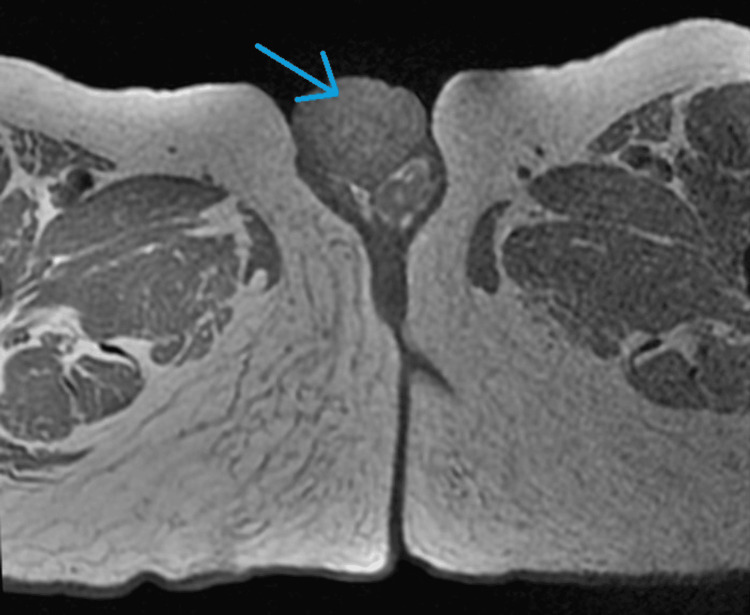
Axial pelvic MRI T1 showing tumor thickening centered on the glans of the penis (blue arrow)
